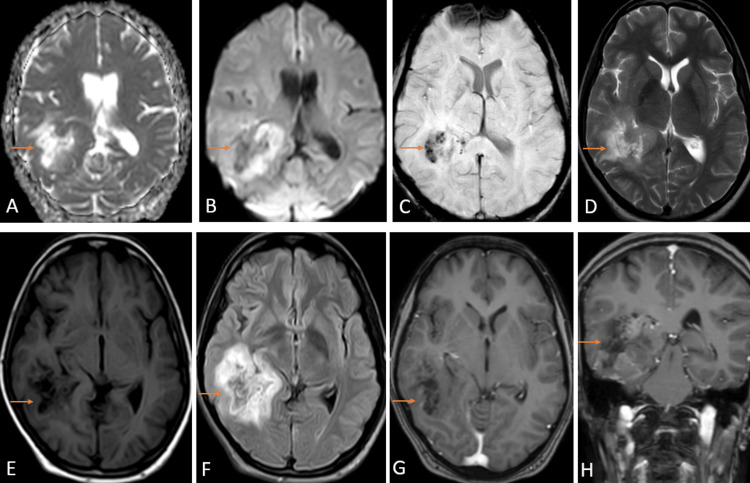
MRI brain with contrast axial section of ADC (A), DWI (B), SWI (C), T2WI (D), T1WI (E), FLAIR sequence (F), post contrast T1WI (G), and post contrast T1WI coronal section (H) showing an ill-defined, thick-walled, intraaxial, minimally enhancing altered signal intensity lesion with perilesional edema in the subcortical and deep white matter with overlying thickened cortex in the right parietal and temporal region. The lesion shows peripheral restriction on DWI with corresponding low signal intensity on ADC, appearing heterogeneously hyperintense on T2WI and showing T2IW/FLAIR mismatch, hypointense with small cystic areas on T1WI with few areas of blooming on SWI. DWI: diffusion-weighted imaging; ADC: apparent diffusion coefficient; T2WI: T2 weighted imaging; T1WI: T1 weighted imaging; FLAIR: fluid attenuation inversion recovery; SWI: susceptibility-weighted imaging.
